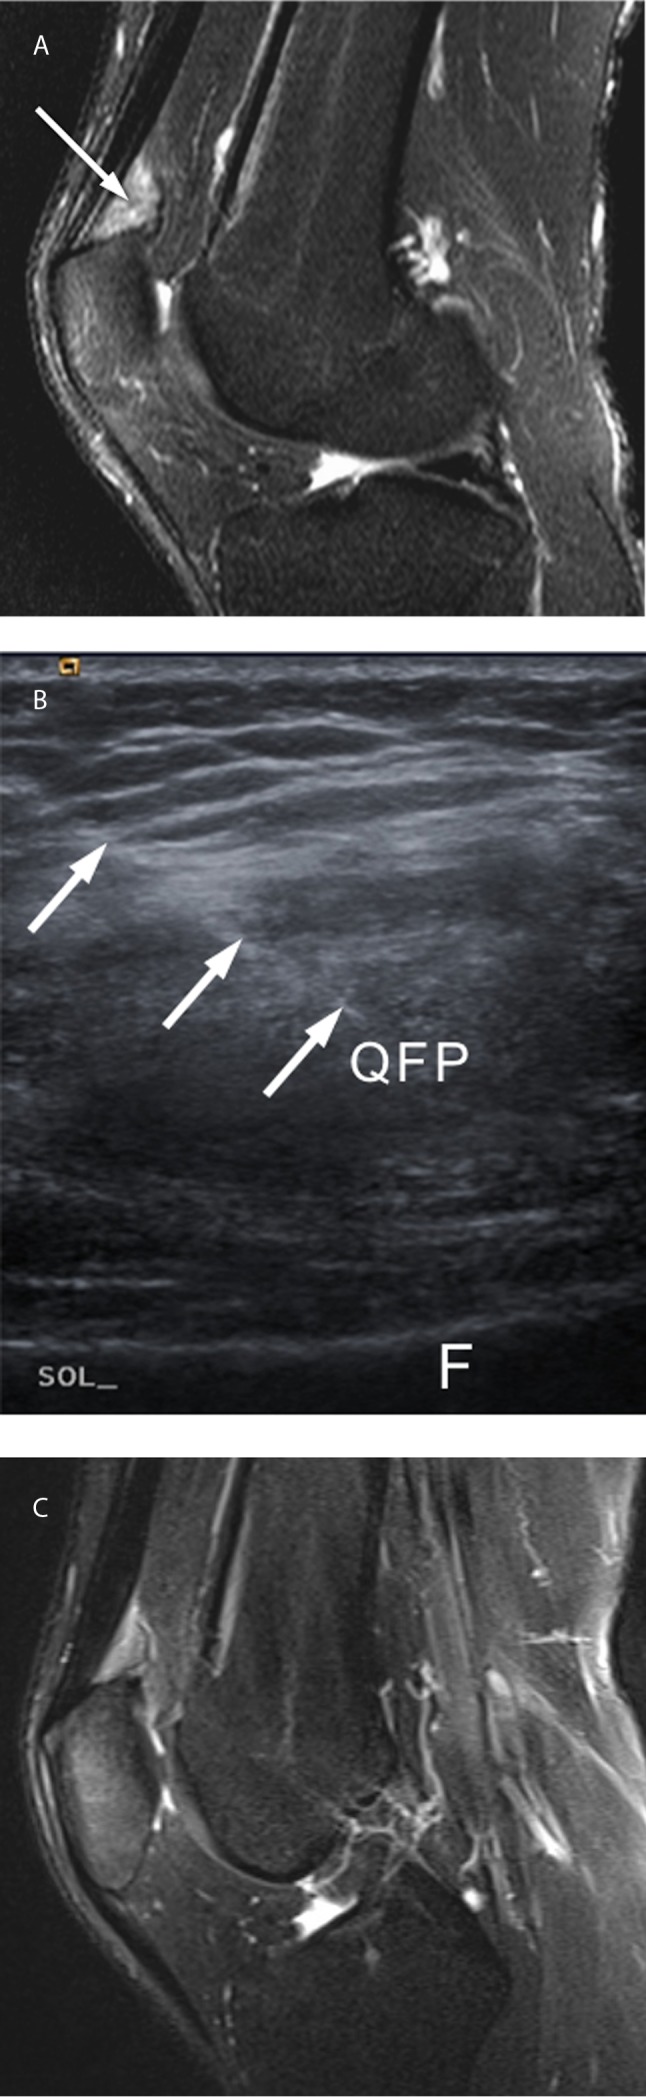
A 31-year-old male with anterior knee pain and suprapatellar tenderness on physical examination. Midsagittal FS T2W (a) MR image (TR/TE, 2728/60 ms) at baseline (Day 0) shows edema-like signal changes with mass effect (posterior bulging) at the quadriceps fat pad *(arrow)*. Oblique parasagittal US image (b) shows the injection needle *(arrow)* traversing through the quadriceps fat pat (QFP) (F, femur). Midsagittal FS T2W (c) MR image (TR/TE, 4020/64 ms) at 6-month follow-up (Day 180) shows persistence of edema-like signal changes at the quadriceps fat pad.
